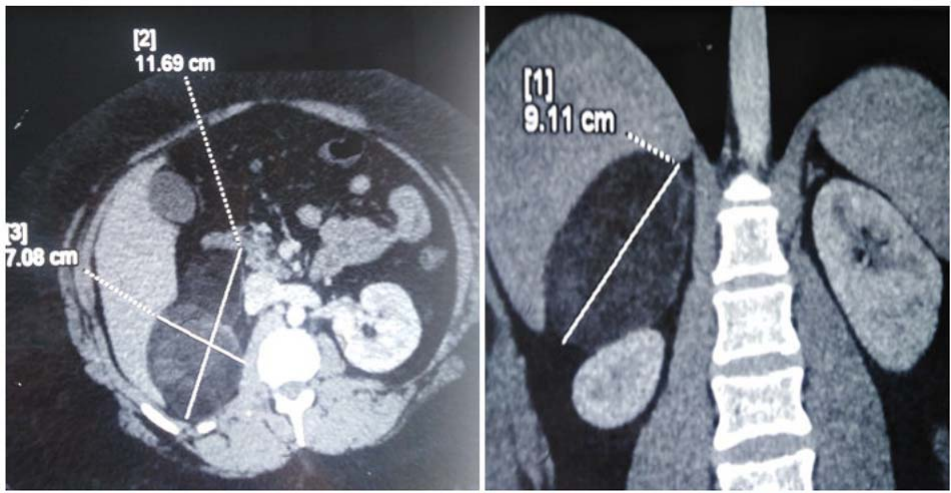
CT scan image showing right adrenal fatty mass.
